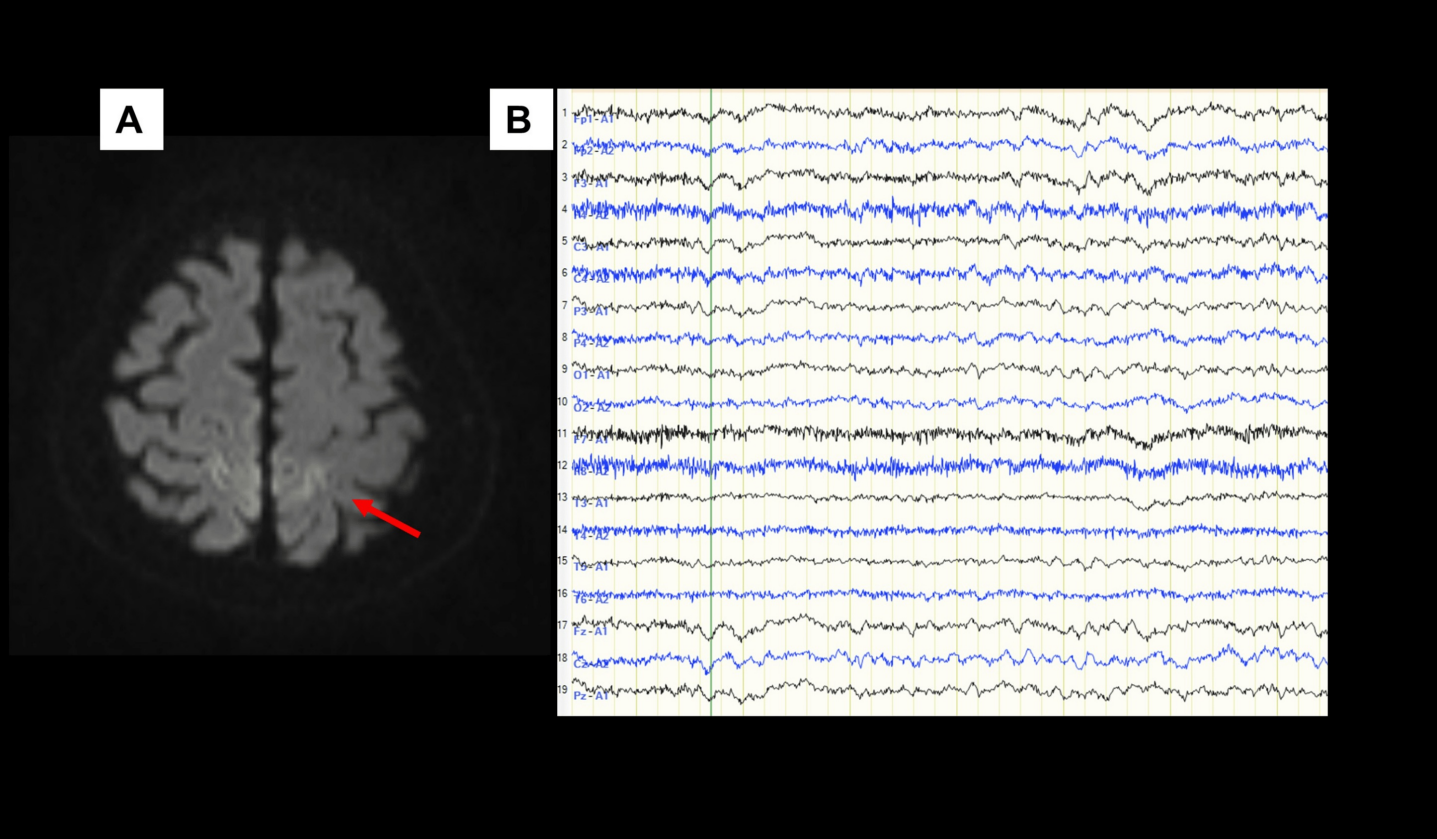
Initial MRI and EEG of the patient (A) Diffusion-weighted brain MRI performed in mid-July (axial view) suggested high signal intensity in the parietal lobe (red arrow), but it was considered to be an artifact caused by a body movement. (B) There were no notable abnormalities on the EEG in mid-August.
